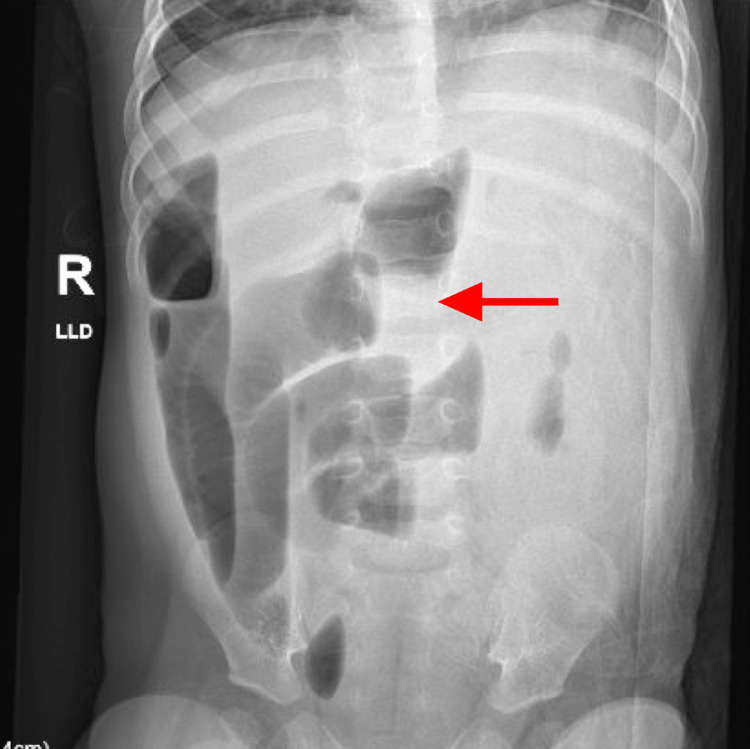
X-ray of the abdomen: AP view Numerous abnormally dilated gas and fluid distention loops of the small bowel throughout the abdomen consistent with small bowel obstruction AP: anteroposterior
